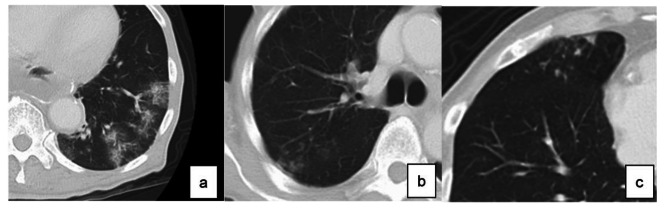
Definition of the diagnosis of pneumonia based on CT consolidation type, b. reticular type, c. nodular type
